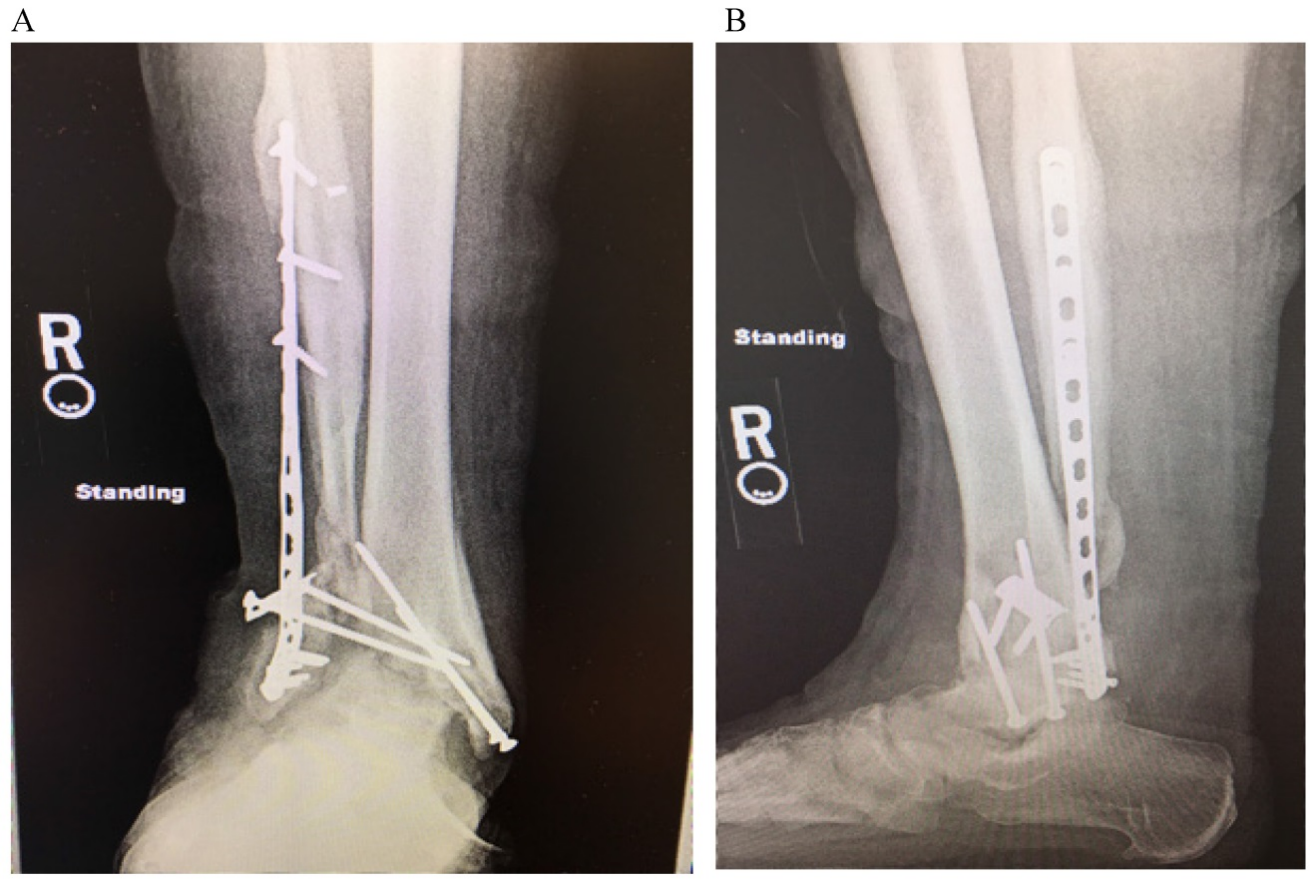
AP (A) and lateral (B) radiograph demonstrating hardware failure and destruction of the tibiotalar joint with severe valgus deformity and non-union
